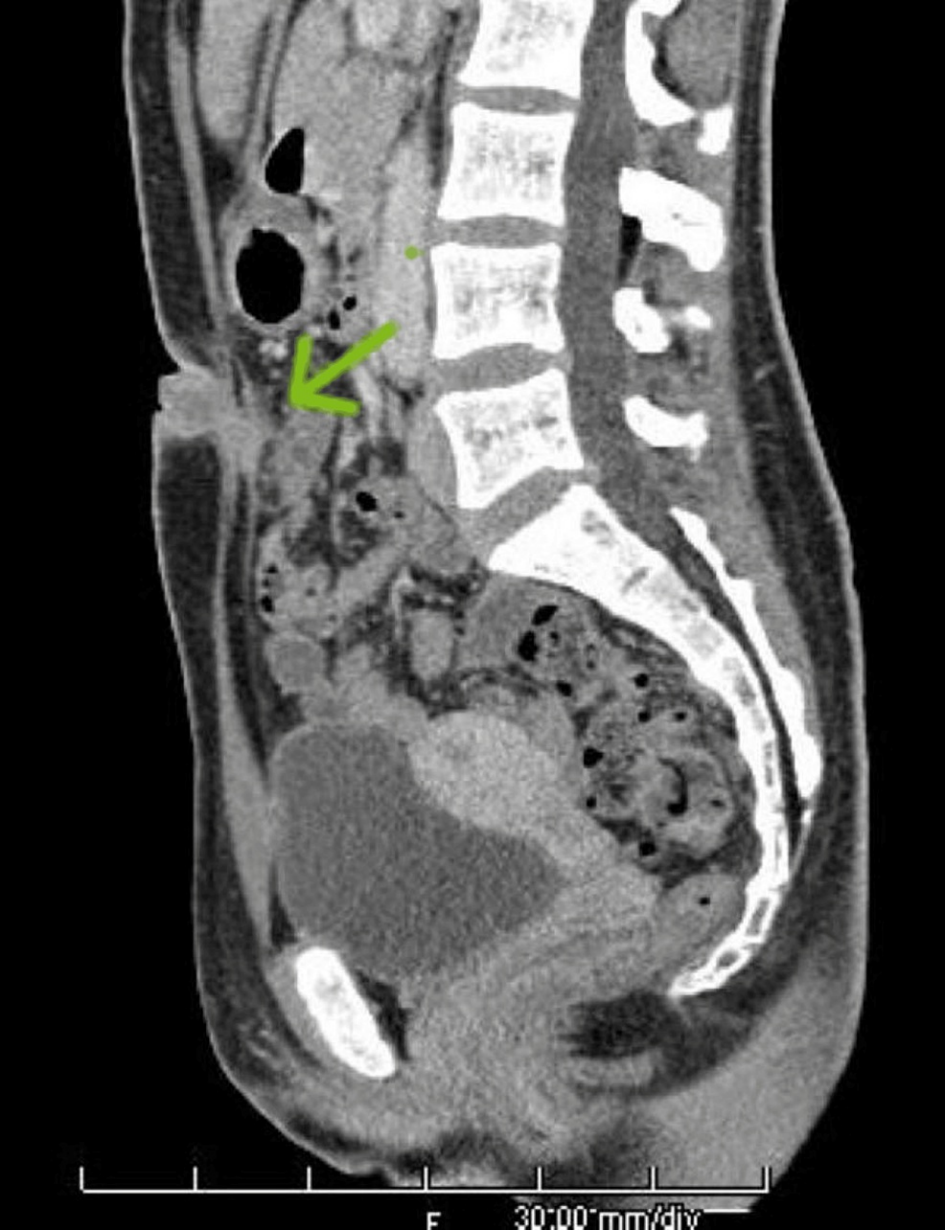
Contrast CT findings at the first visit: sagittal section. The arrow indicates the urachal remnant of the umbilical urachal sinus type and internal poor granulation.
